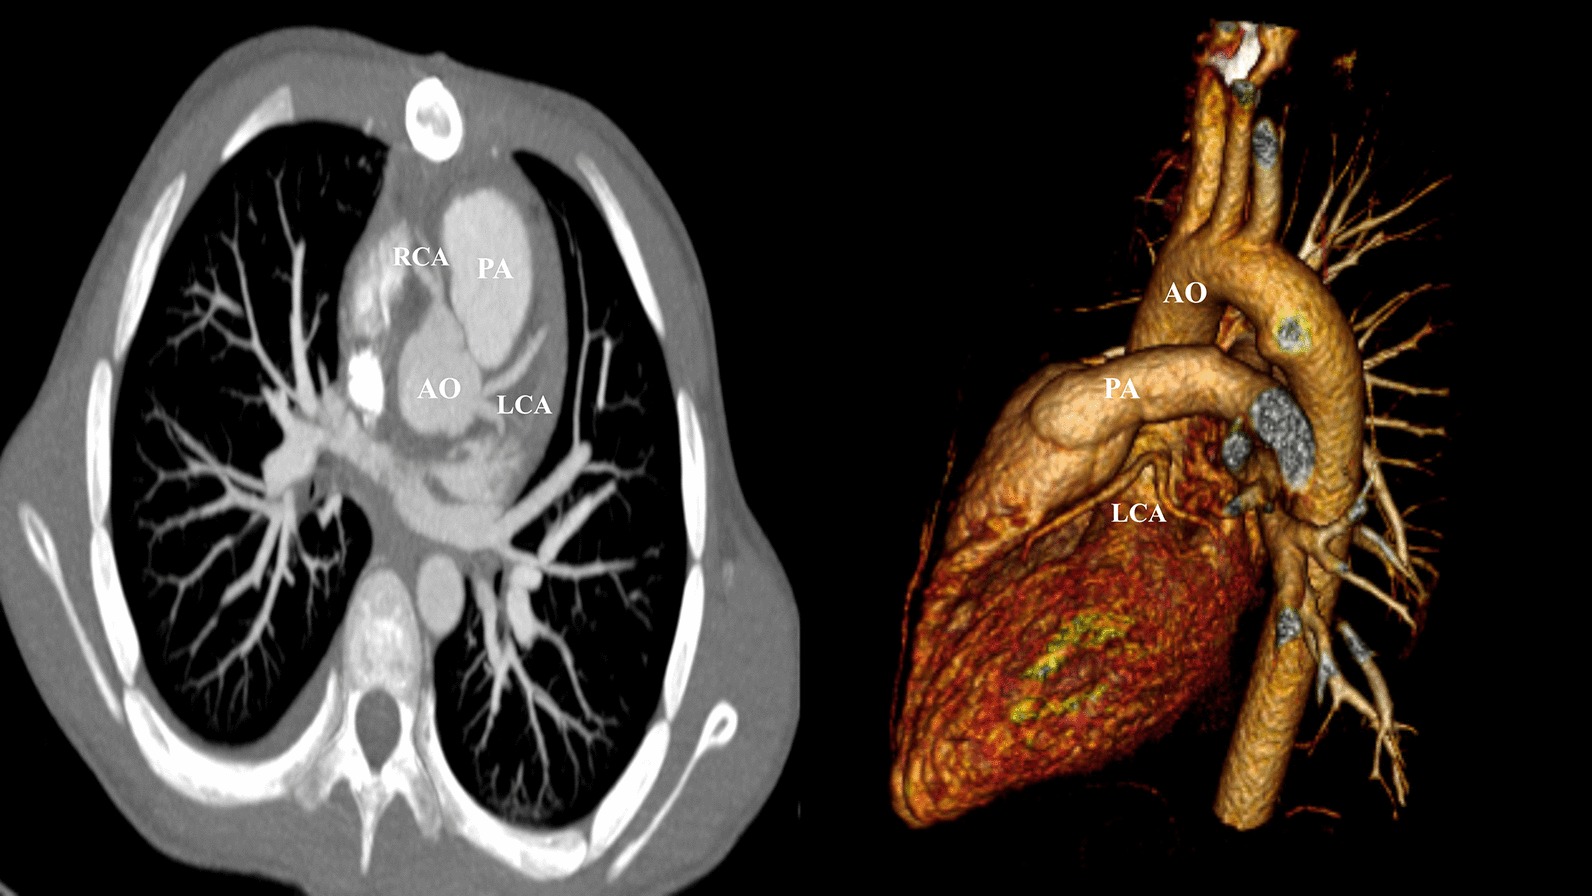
Postoperative CT revealed no stenosis of LCA and no interarterial compression
